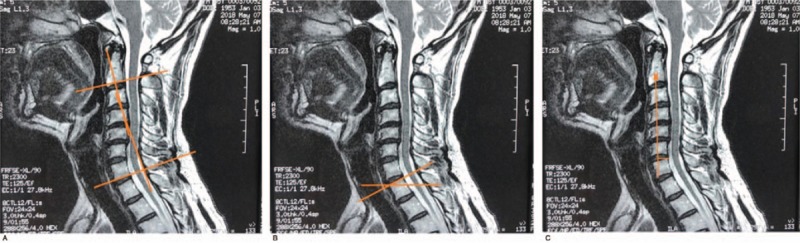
Preoperative neutral sagittal view of T2-weighted image in 2018. (A) showed C2–C7 Cobb angle; (B) showed the angle of T1 slope; (C) showed C2–C7 sagittal vertical axis.
